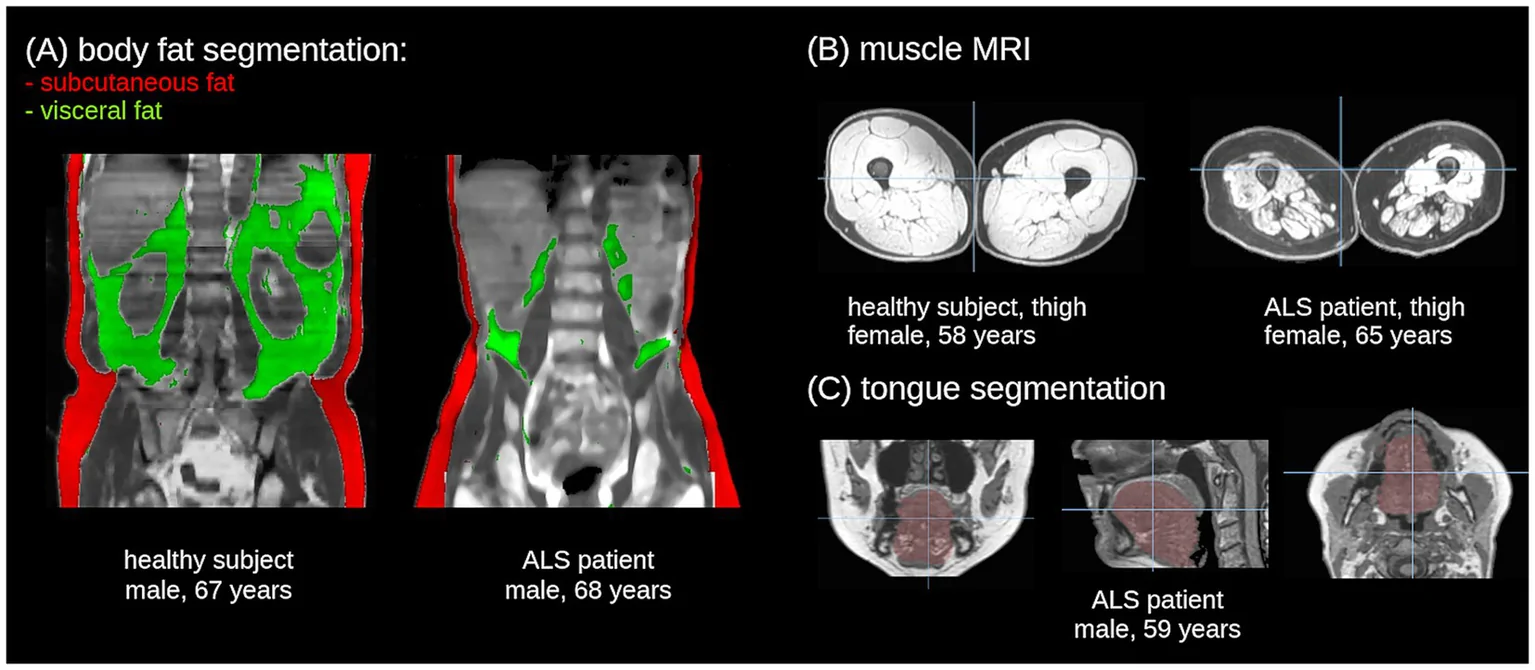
Examples for extra-CNS MRI application in ALS. (A) Body fat segmentation (subcutaneous and visceral fat compartments) could be performed from standard T1w. Left image: Healthy subject with body mass index (BMI) of 27, right image, ALS patient with BMI of 19. (B) Muscle MRI from a Dixon technique shows differences between healthy subjects and ALS patients with respect to atrophy and signal changes. (C) Tongue segmentation could be performed from T1w MRI (MPRAGE).
